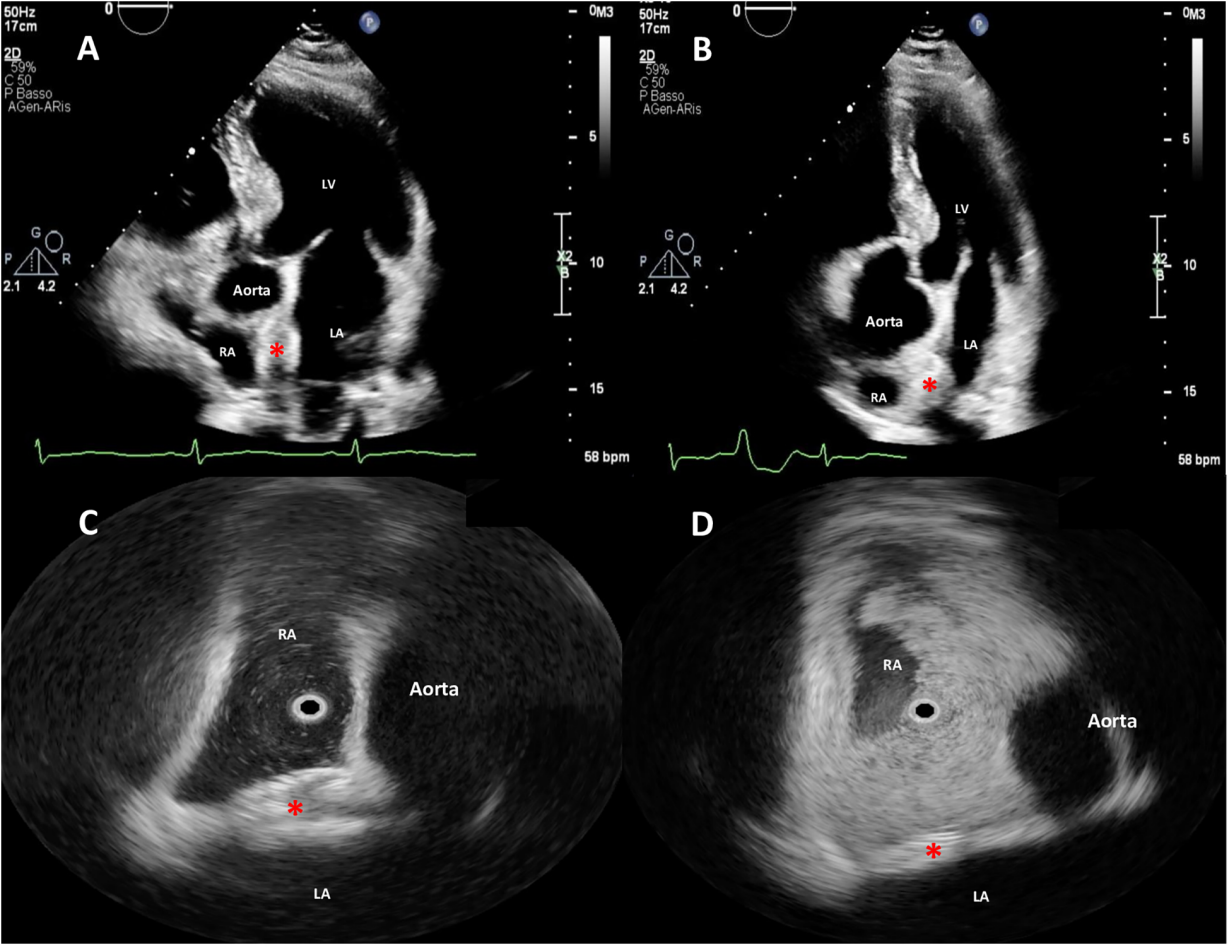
Two-dimensional (2D) transthoracic echocardiography (TTE) color Doppler (A,B) and rotational intracardiac echocardiography (rICE) (C,D) images demonstrating the final correct position of the PFO device (red asterisks), parallel to interatrial septum (IAS), not impinging the aortic wall, without residual shunt after agitated saline injection. RA, right atrium; LA, left atrium; LV, left ventricle.
